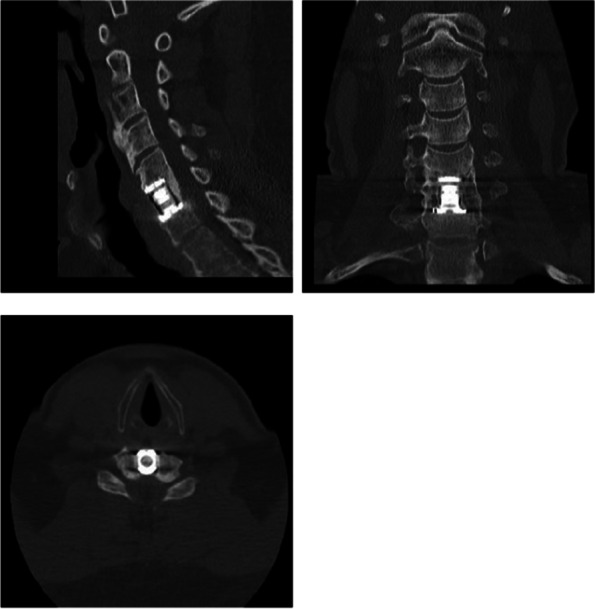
A CT scan at final follow-up shows fusion with presence of bone mass inside the cage
